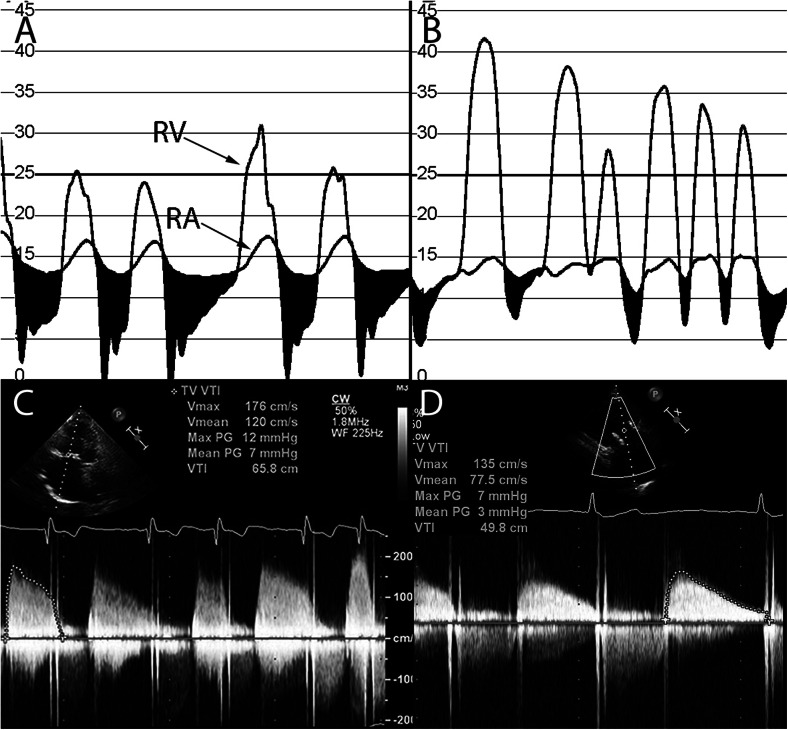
a Invasive hemodynamic tracings of simultaneous right ventricular and right atrial pressures in a patient with atrial fibrillation and a stenotic bioprosthetic tricuspid valve showing elevated diastolic gradients (*shaded black area*)—mean gradient 8 mmHg. b Invasive hemodynamic tracings of right ventricular and right atrial pressures showing reduction of tricuspid stenosis to the mild range (mean gradient 3 mmHg) after transcutaneous valve placement. c Transthoracic echocardiographic image with CW Doppler across the tricuspid valve showing moderate stenosis (mean gradient 7 mmHg). d Transthoracic echocardiographic image with CW Doppler across the tricuspid valve demonstrating reduction of mean gradient to 3 mmHg after transcutaneous valve placement
